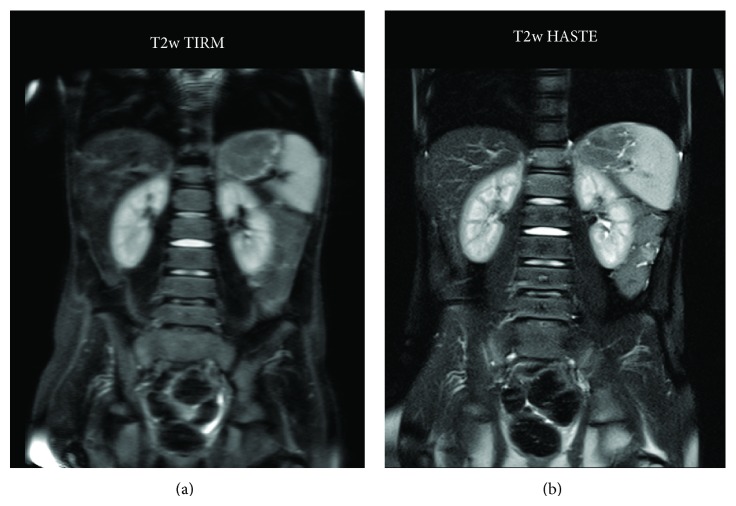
Coronal T2w TIRM (a) and high-resolution T2w HASTE FS (b) imaging in an 8-year-old girl with chronic abdominal pain and normal abdominal ultrasonography. In the absence of any pathological findings, the TIRM image, owing to the long repetition time, is considerably blurred by motion artefacts in the restless and continuously shifting patient. Repeating the T2 TIRM sequence did not improve image quality. HASTE imaging does not show any motion artefacts and clearly depicts bony structures including the lower thoracic and lumbal spine as well as the abdominal organs. Incomplete saturation of fatty tissue signal towards lower image margin is more noticeable with HASTE than with TIRM imaging. Image quality was graded as IQ = 2 (TIRM) and IQ = 4 (HASTE).
